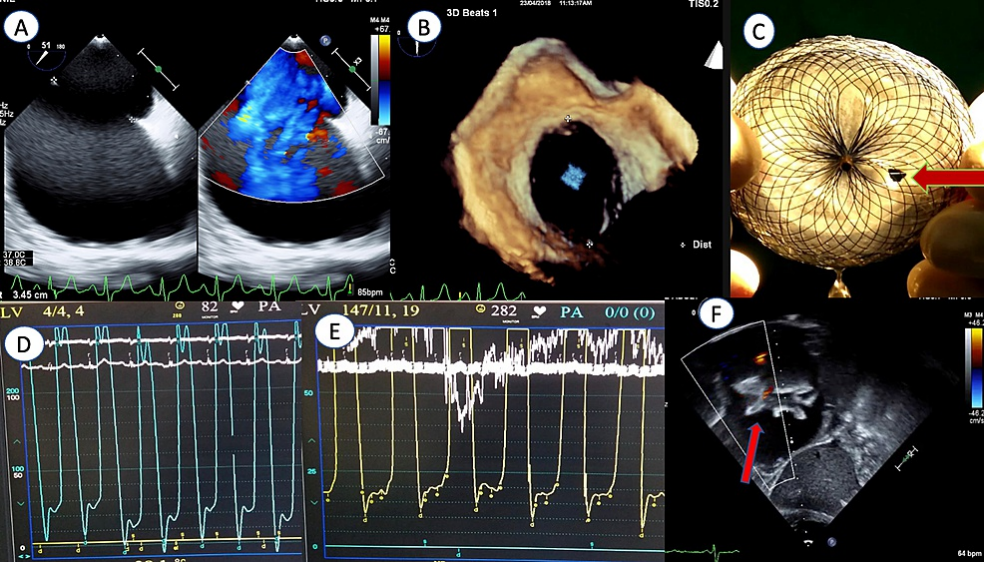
(a) Transesophageal echocardiography (TEE) with color Doppler showing very large atrial septal defect with left-to-right shunt. (b) Three-dimensional TEE large defect. (c) Fenestration in the atrial septal occluder (arrow). (d) Basal left ventricular end-diastolic pressure (100 scales). (e) Post balloon occlusion left ventricular end-diastolic pressures (50 scales). (f) Post device closure transthoracic echocardiogram in subcostal view showing good device position and fenestration flow left-to-right (arrow).
